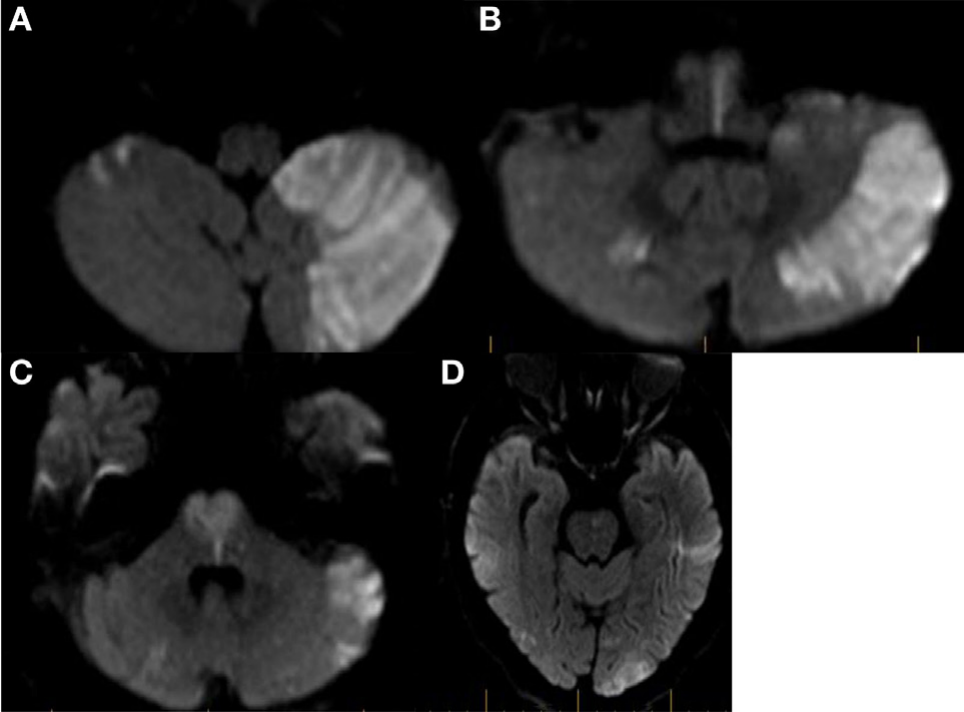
Example of Bern diffusion-weighted magnetic resonance imaging (DWI) and posterior circulation acute stroke prognosis early CT score (pc-ASPECTS) calculation. (A) Axial diffusion-weighted MRI in a patient with acute basilar artery occlusion. Restricted diffusion is seen in both cerebellar hemispheres. In the left cerebellar hemisphere >1/3 of the territory is involved. 3 points are assigned for the Bern DWI score and 2 points are assigned for the pcASPECTS score. (B) Restricted diffusion is noted involving bilateral pyramidal tracts at the level of the medulla. 4 points are assigned to the Bern DWI score and 0 points are assigned to the pc-ASPECTS. (C) Restricted diffusion is noted involving bilateral corticospinal tracts at the level of the pons. 4 points are assigned to the Bern DWI score and 2 points are assigned to the pcASPECTS score. (D) Restricted diffusion is noted in bilateral temporo-occipital regions. A score of 2 is assigned to both the Bern DWI score and the pcASPECTS score. Total Bern DWI score is 13. Total pcASPECTS score is 4.
